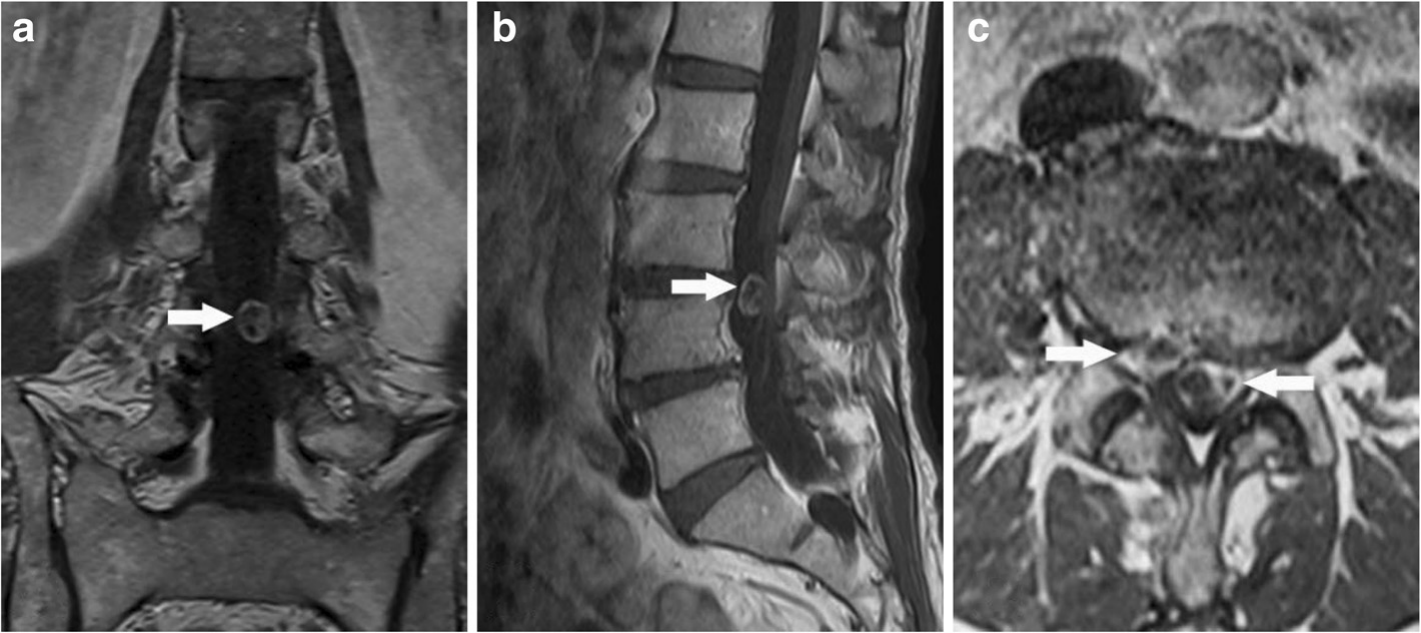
Gadolinium contrast MR images (a coronal image, b sagittal image) demonstrated a partially enhanced intradural mass at the left side of the L3/4 spinal canal. Axial image (c) showed an intradural mass of heterogeneous signal at the left and a herniated disc at the right extradural space
